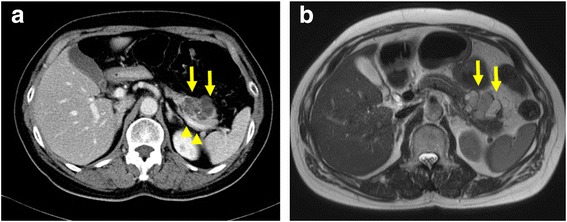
Findings from preoperative CT and MRI. CT and MRI show a cystic neoplasm (*arrow*) in the pancreatic body. Cyst diameter is 35 mm. a CT. b T2-weighted MRI. Splenic vein was normal status (*arrowhead*)
